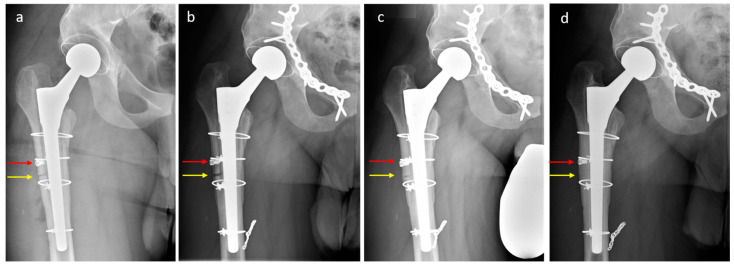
Radiographs 3 days (a), 3 months (b), 6 months (c), and 18 months (d) postoperatively documenting the progressive bony union of the osteotomy without dislocation (in the interim, the patient received osteosynthesis of the anterior acetabular column for symptomatic non-union after a previous periacetabular osteotomy and coiling of a pseudoaneurysm of a branch of the profunda femoris artery). Note the position of the screw (red arrow) and the gradual healing of the osteotomy site (yellow arrow).
